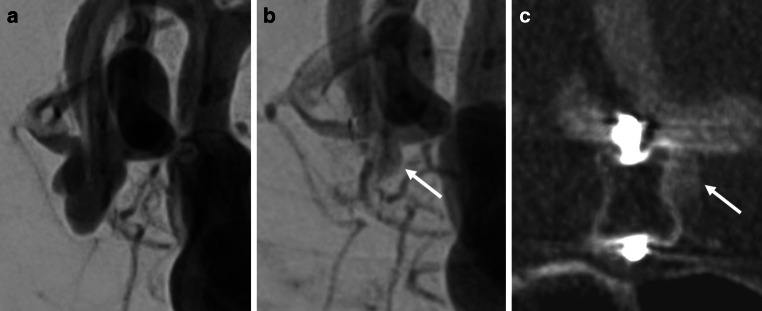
A representative case of an anterior communicating artery aneurysm treated using the Woven EndoBridge (WEB) device. a Digital subtraction angiography (DSA) prior to WEB placement. (b) DSA and (c) intravenous cone-beam computed tomography (IVCBCT) at the 6‑month follow-up showing an aneurysm remnant outside of the WEB device (white arrows) classified as BOSS 3
